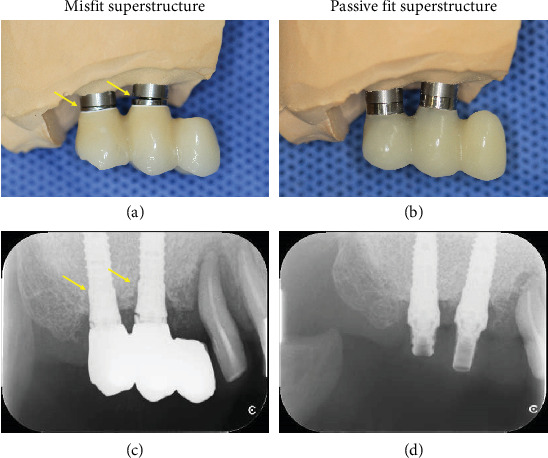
Fit evaluation of the superstructure using an intraoral working cast model and radiograph analysis (yellow arrows indicate micro gap). (a) Image showing the misfits between the previously fabricated superstructure and the abutment analog. (b) Image showing the fit between the resin-based provisional superstructure and the abutment analog. (c) Radiograph showing misfits of the previously fabricated superstructure and the abutment level of the subject. (d) Radiograph showing an almost perfect fit between the fabricated provisional superstructure and the abutment level of the subject.
